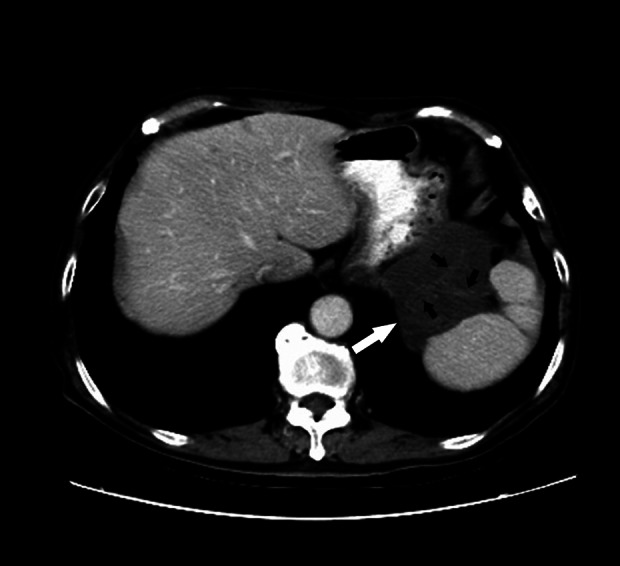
Transaxial enhanced abdominal CT scan showing an unenhanced gyrus-like mass (white arrow) which was divided into 3 parts by linear stripes (black arrow; ‘ribbon sign’).
